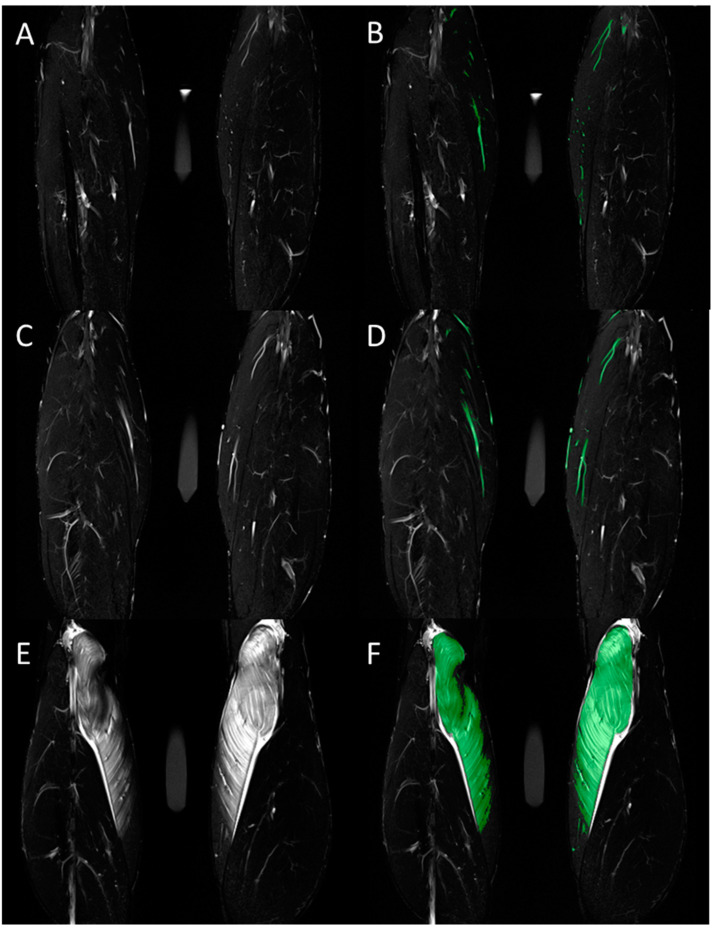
Total volume of intramuscular edema was assessed in the coronal T2-weighted TIRM images. Representative images before (A,C,E) and after (B,D,F) color-coded manual segmentation of the edematous medial gastrocnemius muscle are shown for measurements at baseline (A,B) and at 6 h (C,D) and 48 h (E,F) after standardized exercise. Hyperintense vessels were segmented in the baseline images and subtracted from the segmented volume in the post-exercise images.
